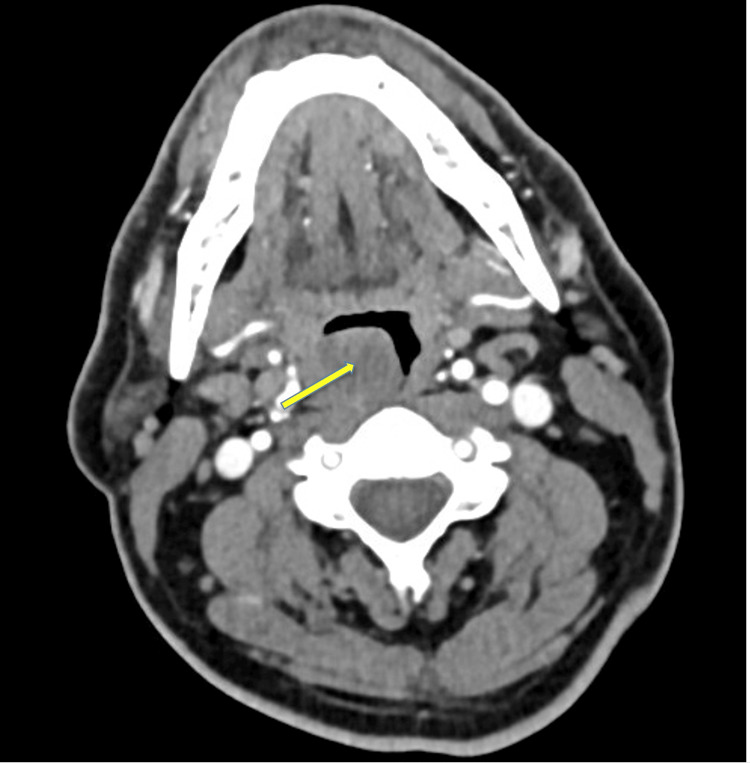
CT of the neck showing the mass
